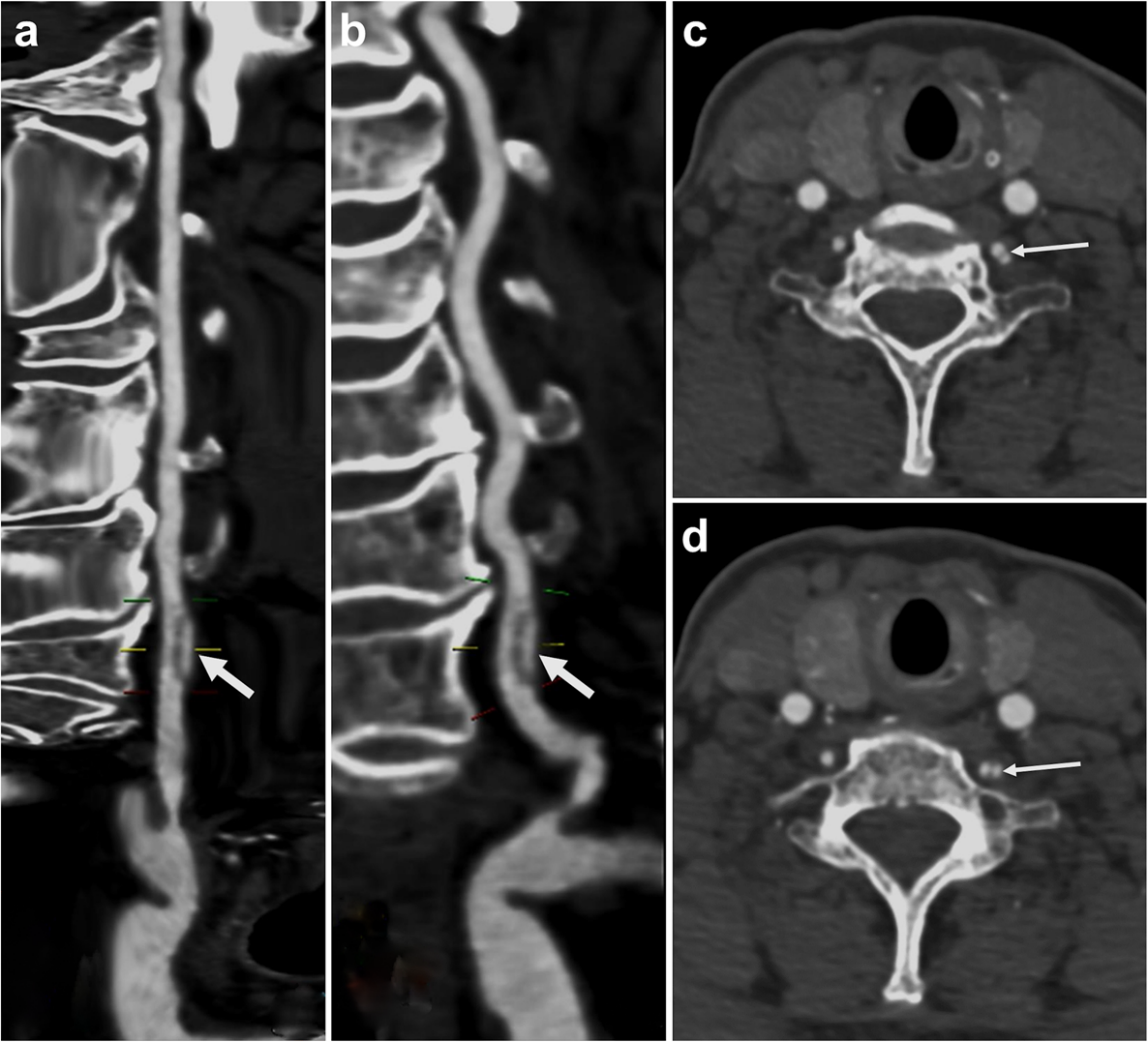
The ‘double lumen’ sign and ‘intimal flap’ in CTA. CTA reconstruction images showing the ‘double lumen’ appearance at the V1 segment of the left vertebral artery (thick white arrows) (a-b). CTA axial images showing ‘double lumen’ sign and ‘intimal flap’ (thin white arrows) (c-d). CTA = Computed tomography angiography
